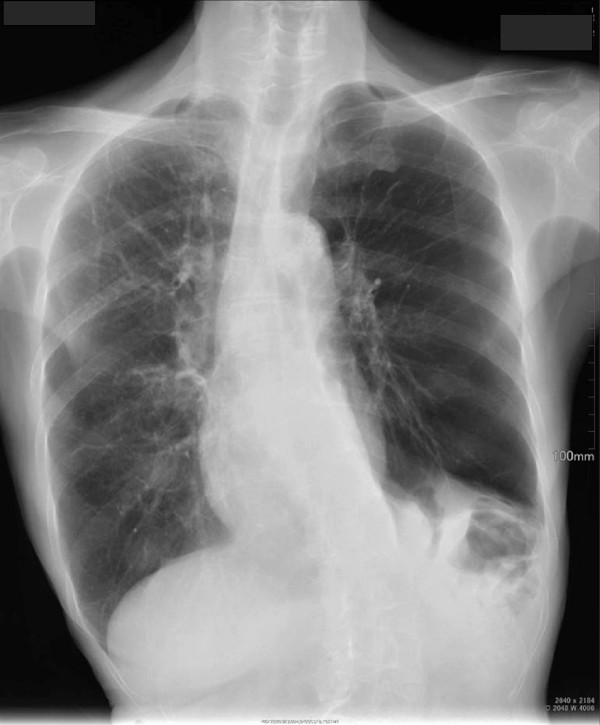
Chest roentgenography on admission showing multiple bullae and bilateral emphysema with left diaphragmatic hernia.
